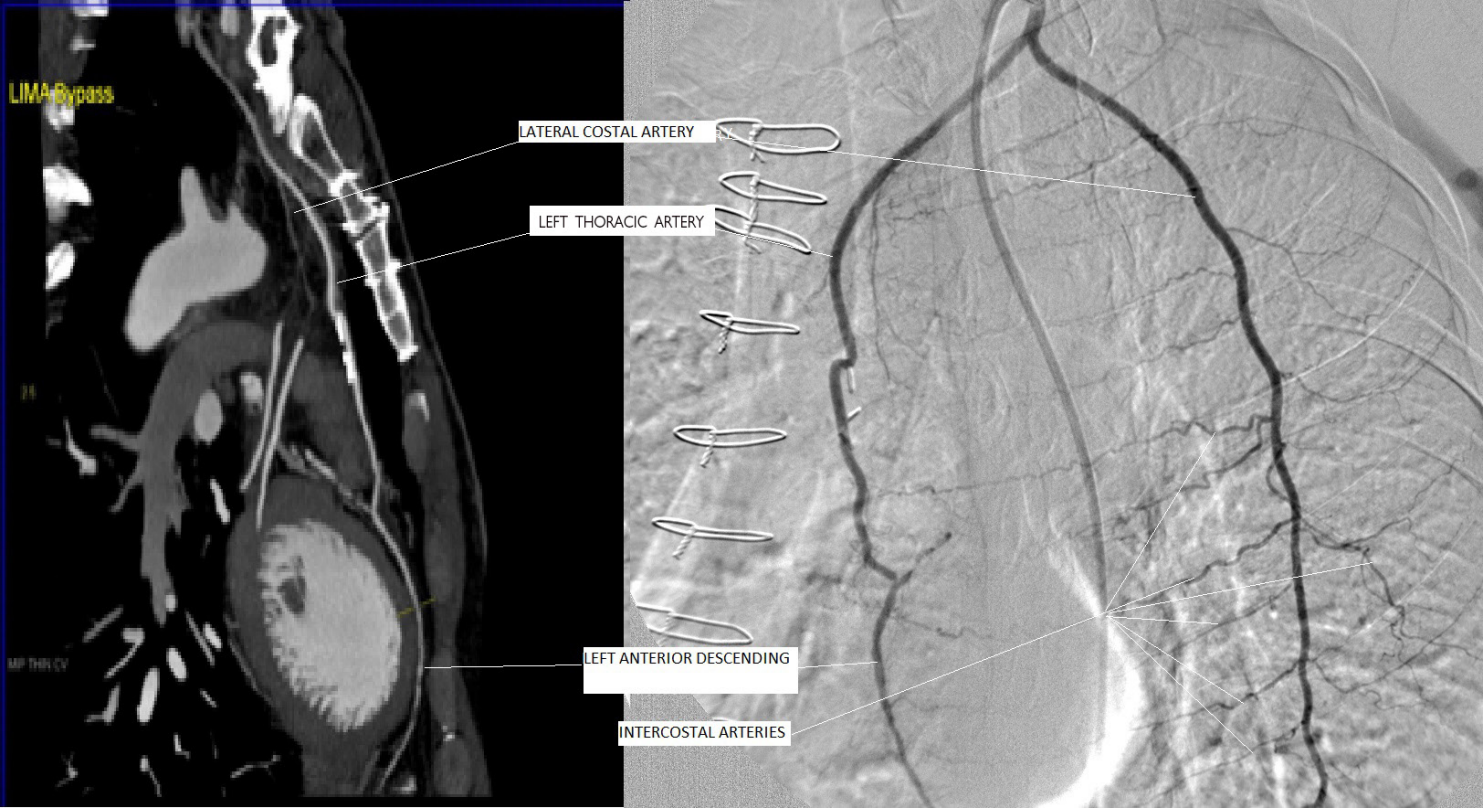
Computed tomography e cineangiographic view of the undivided LCA branch
